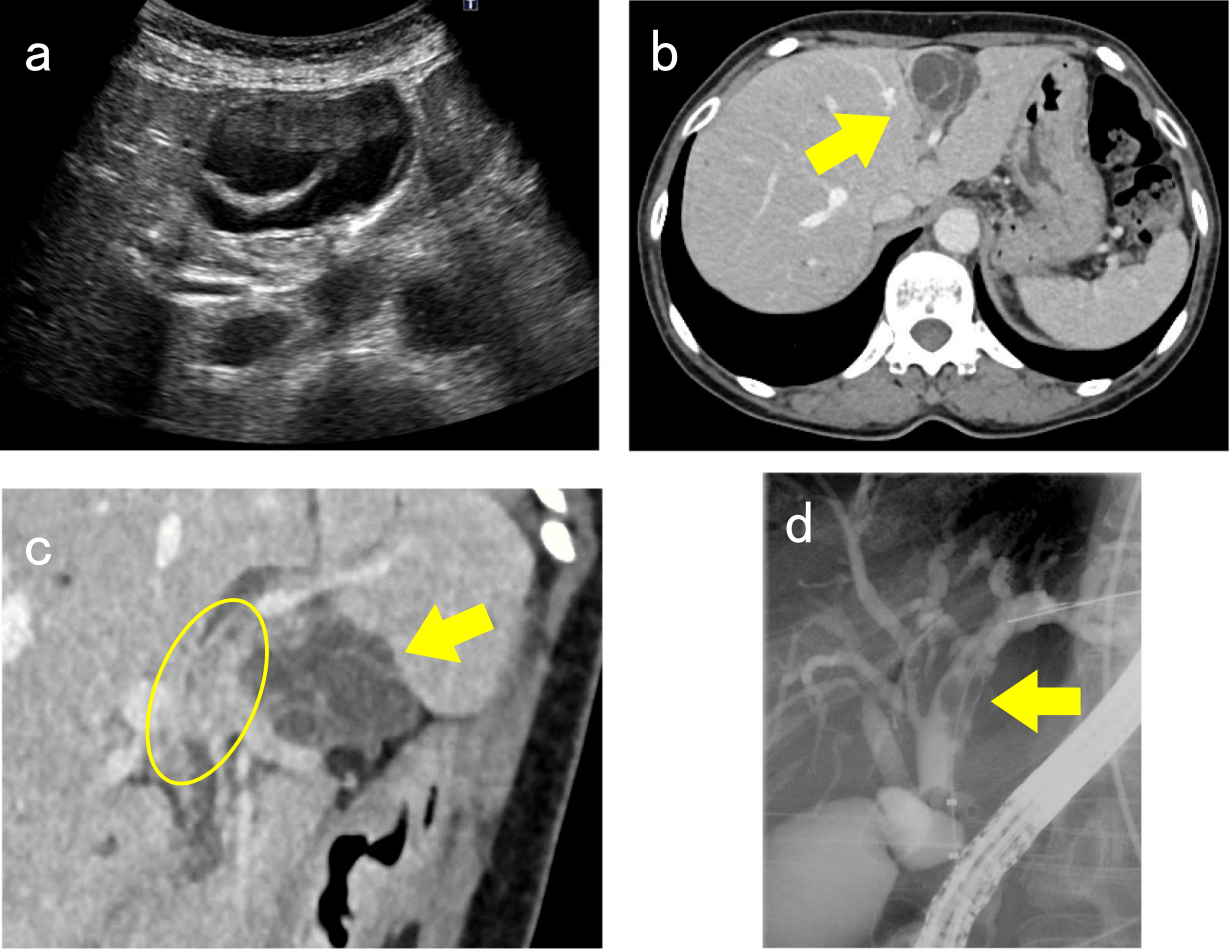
Imaging examination findings. a Abdominal ultrasonography showed a 49 × 29-mm cystic lesion with a septum in Segment 4 (S4). b Computed tomography (CT) revealed a 4-cm multilocular cystic lesion with multiple septal formations showing a cyst-in-cyst appearance in S4 (arrow). c CT revealed polypoid nodule (oval) protruded in the left hepatic duct from the cystic lesion (arrow). d Endoscopic retrograde cholangiography showed a contrast defect in the left hepatic duct to common bile duct which was suspected to be a nodular lesion in the bile duct (arrow)
